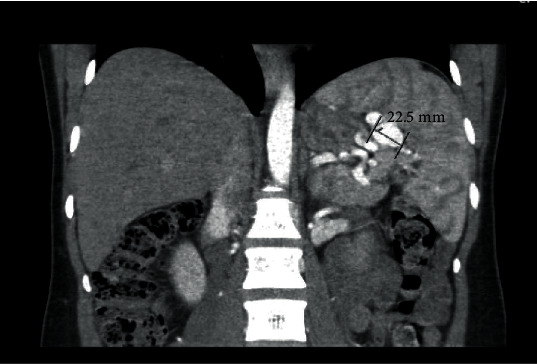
CT abdomen with contrast showing the splenic artery aneurysm (reproduced from Meghan et al. [9], [under the Creative Commons Attribution License/public domain]).
